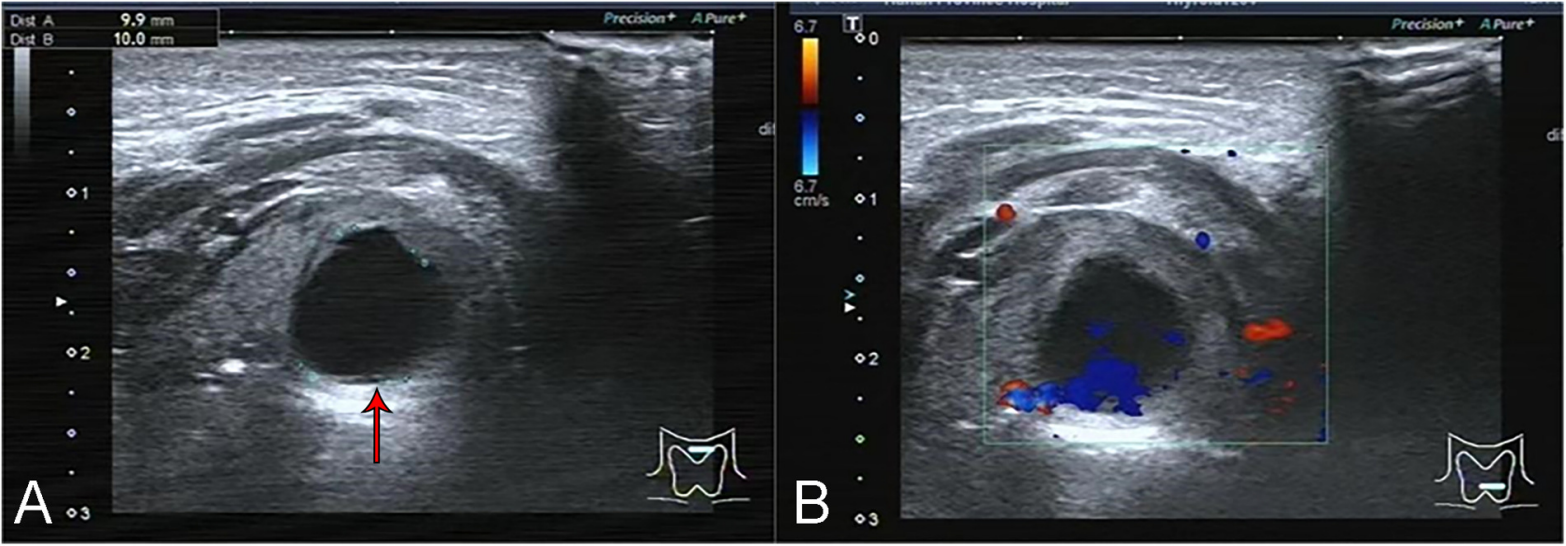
Ultrasound images of the neck of the 19th child in table 2. (A) The cyst appeared on ultrasound as a 9.9 × 10.0 mm anechoic zone with clear borders. (B) There was no blood flow signal in the cyst.
